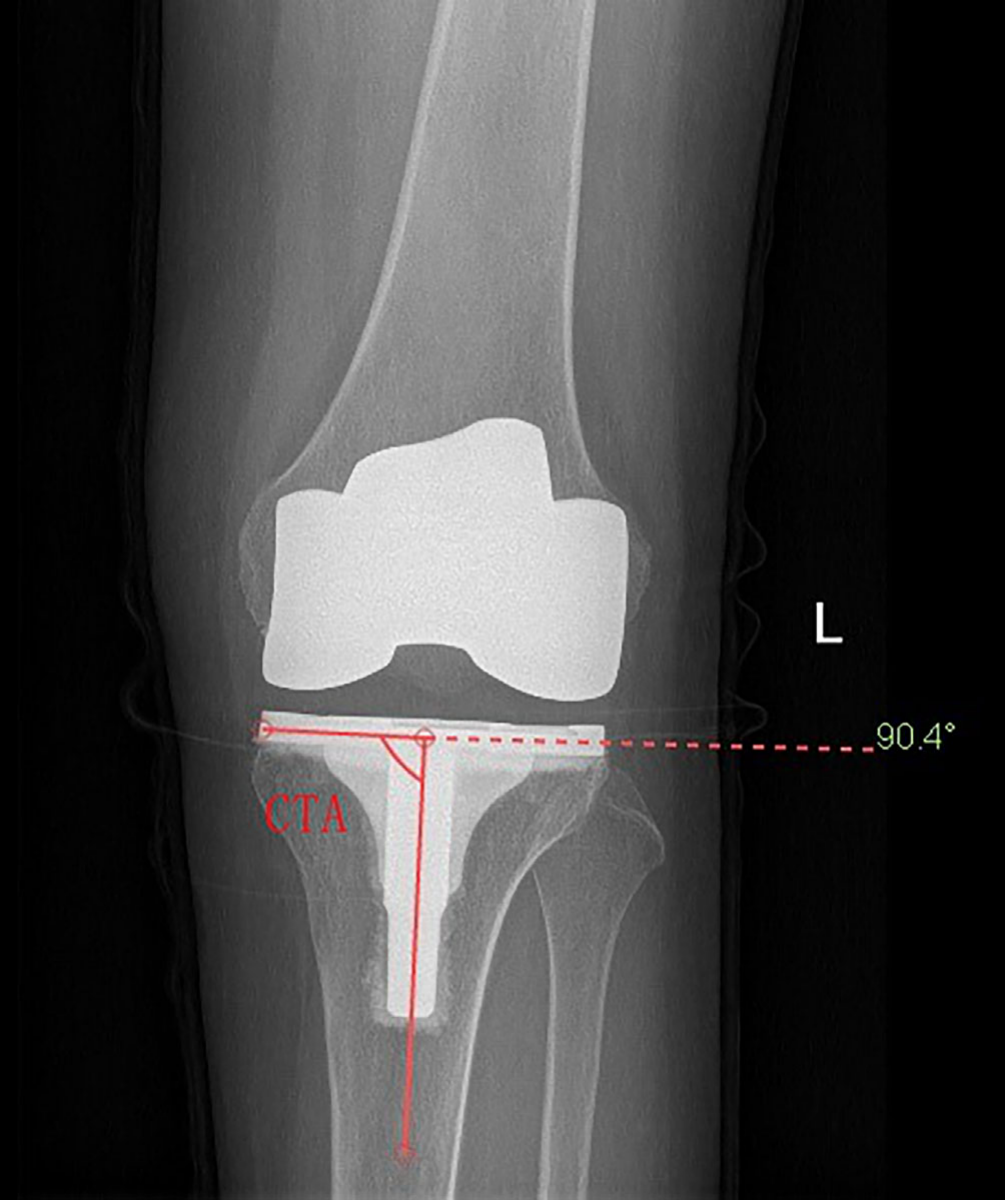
The coronal tibial component angle (CTA) is the medial angle between the mechanical axis of the tibia and the horizontal axis of the tibial tray.
